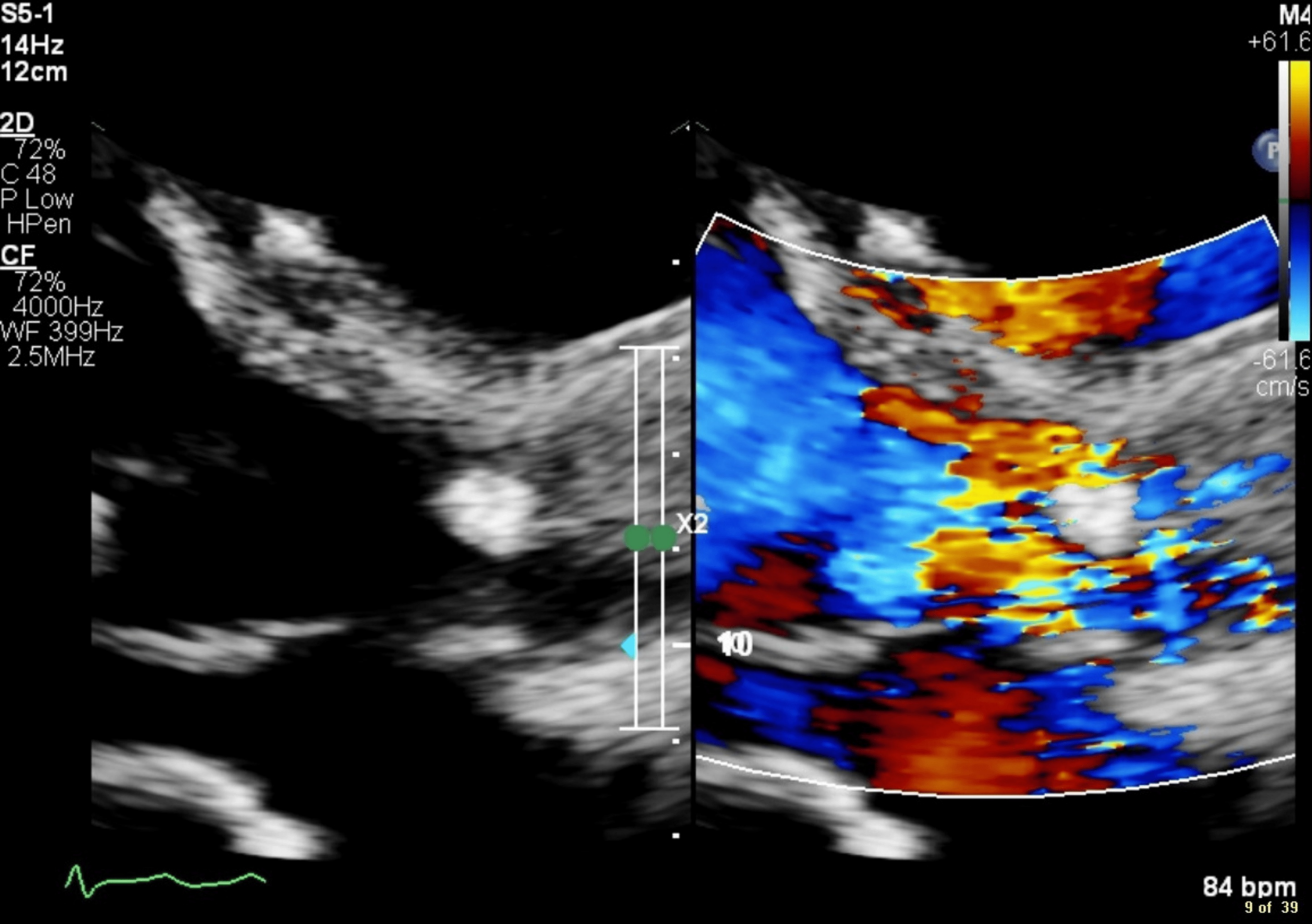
Repeat echocardiogram showing the aortic valve vegetation
